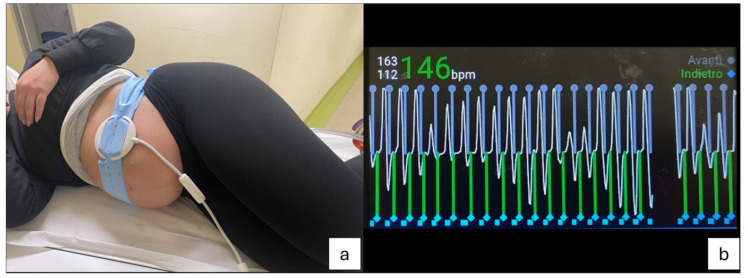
(a) Doppler ultrasound device positioned and fastened on the maternal abdomen in lateral decubitus and (b) fetal heartbeat track obtained with the DUS method.
